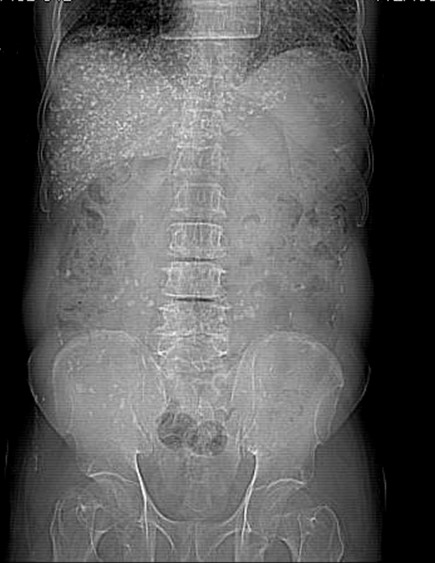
Abdominal CT-scannogram of the same patient showing the numerous comma-shaped calcific densities all over the abdomen, especially over the hepatic region. Degenerative changes are also noted in the lumbar vertebrae
